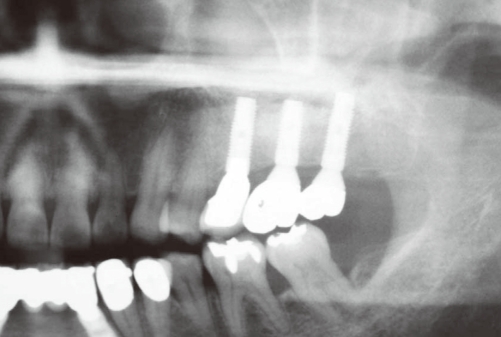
Panoramic radiograph of the dental implants placed in the re-grafted sinus after successfully functioning for 33 months.
